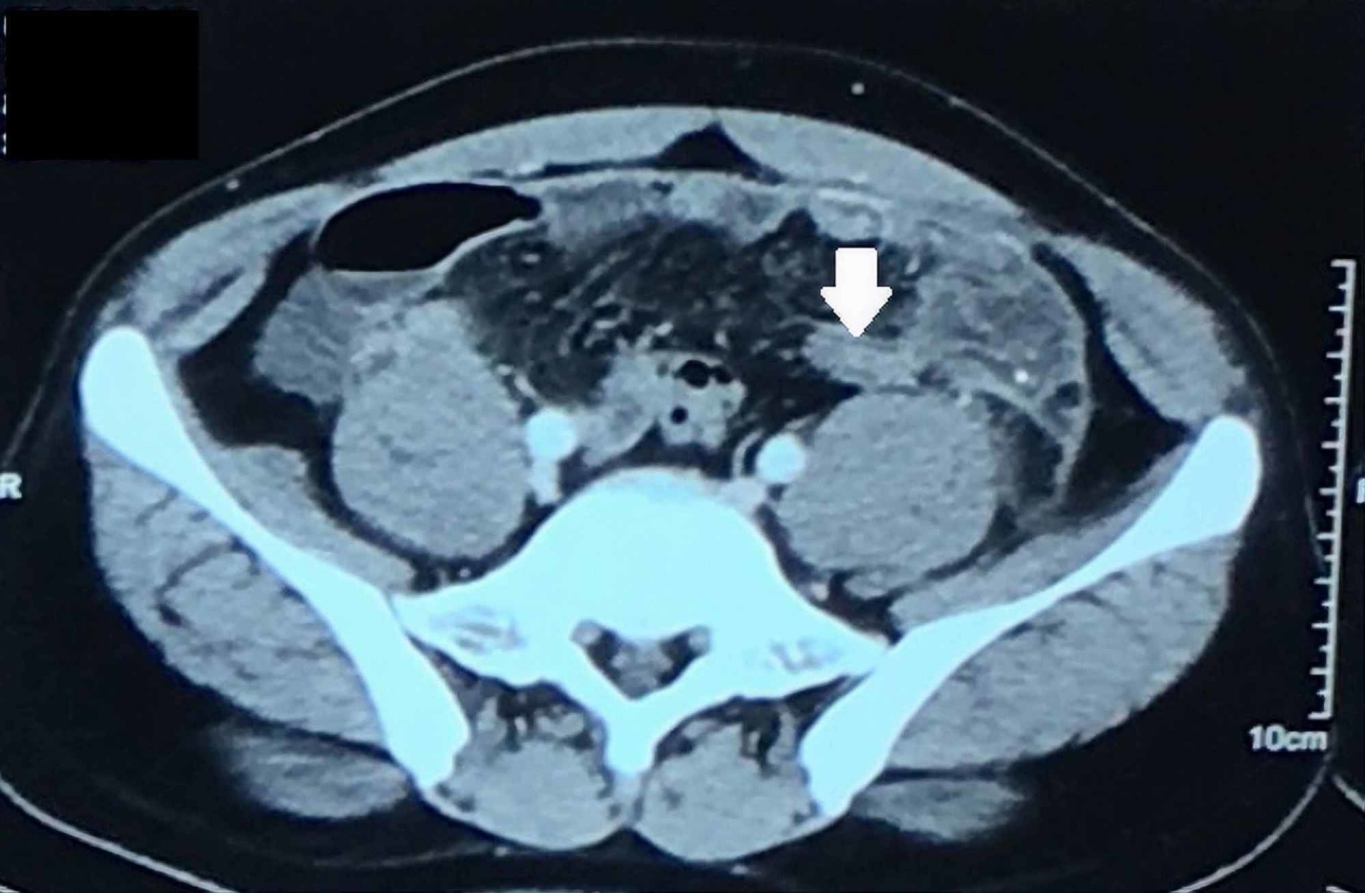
Axial CECT of abdomen and pelvis showing appendix (solid block arrow) arising from the cecum on left iliac fossa with thickened wall and periappendicular fat stranding CECT, contrast-enhanced computed tomography
